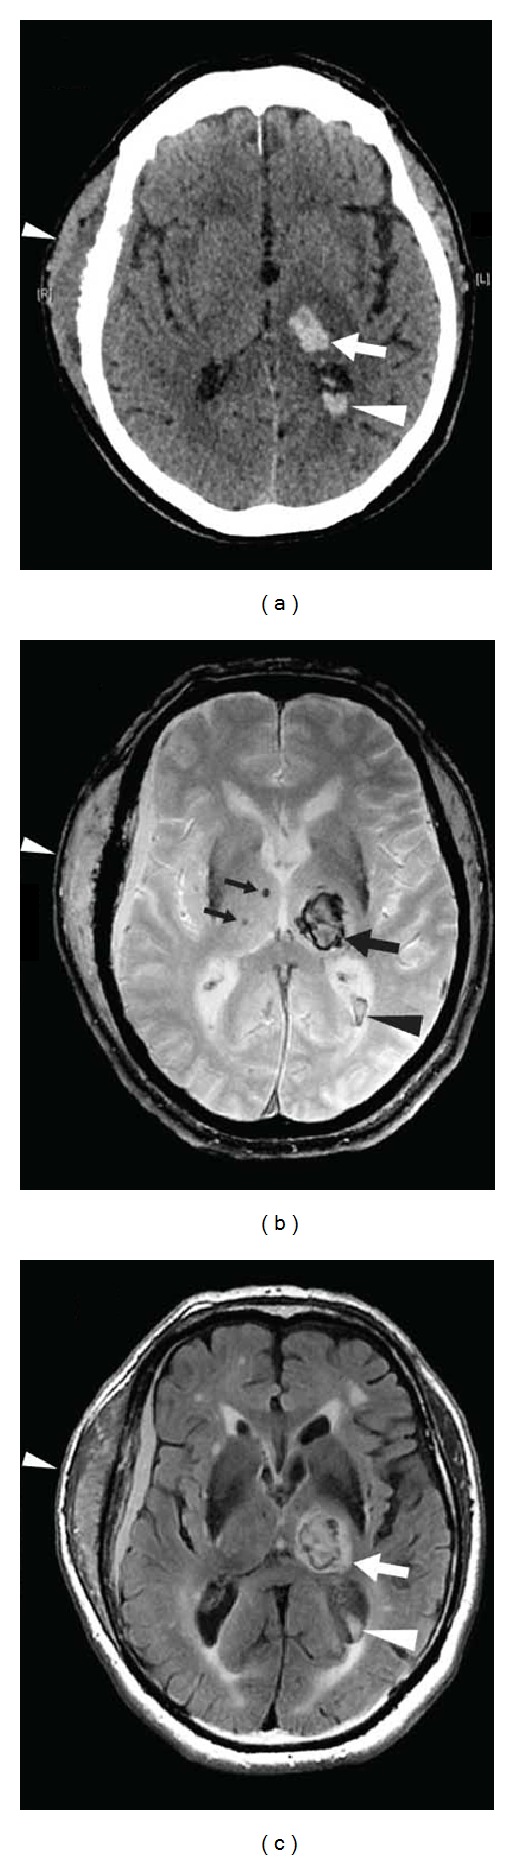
CT scan (a) and gradient-echo-weighted (b) and FLAIR (c) imaging showing a hypertension-related left-sided thalamic hemorrhage (large arrows on a, b, and c) and surrounding edema, complicated with a intraventricular hemorrhage (large arrowheads on a, b, and c) and associated with chronic hypertension-related microbleeds in the right thalamus (small arrows on b) and a subcutaneous and subdural hemorrhage (small arrowheads on a, b, and c) related to trauma (caused by acute right hemiplegie due to the thalamic hemorrhage).
